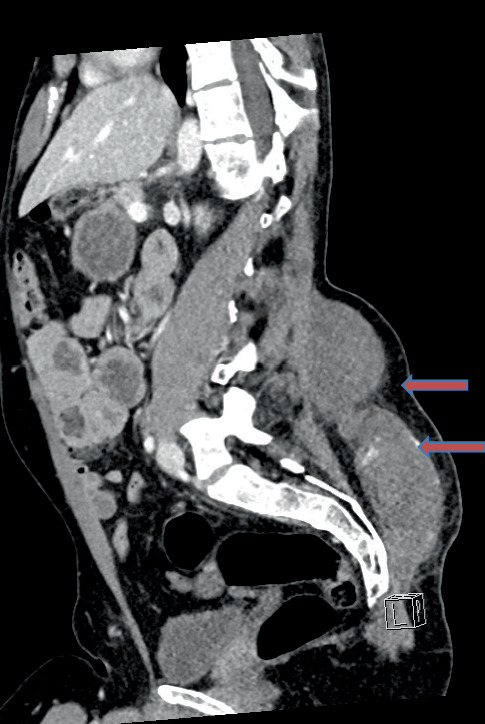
Contrast-enhanced sagittal CT scan of the spine and pelvis on admission, demonstrating a large subcutaneous hematoma in the lumbar region. The hematoma extends cranially from the lumbosacral junction and is associated with multiple sites of contrast extravasation (white areas), indicating ongoing active arterial bleeding
